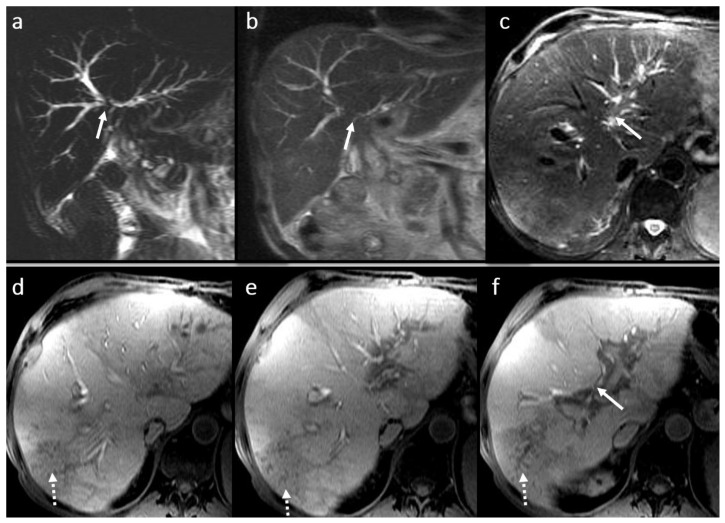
Chronic cholangitis with perianastomotic fibrosis (arrow) in a male patient, 53 years old, 4 year after LT: MRI evaluation in SSFSE long TE (a), SSFSE short TE (b), T2FS wi (c), and 3DT1FS in hepatobiliary phase (d,e): mild dilatation with discrete irregularities and short multiple focal stenosis of the intrahepatic BT secondary to perianastomotic fibrosis; note also small filling defects in the left intrahepatic bile duct (microlithiasis) in association with peripherical pseudo-triangular hypointense T1 liver areas in HBP corresponding to focal liver disfunction due to biliary obstruction (dotted arrow) (f).
